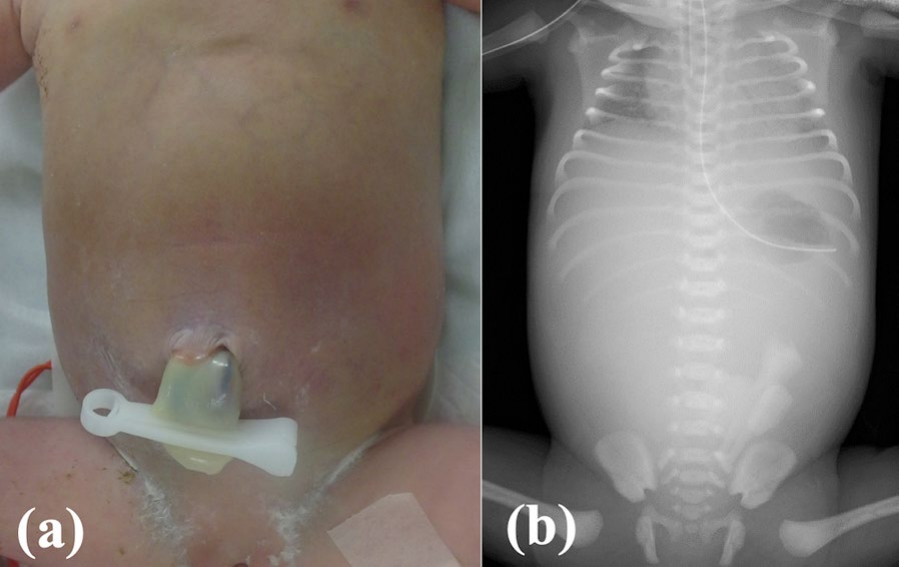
General appearance and the X-ray at birth: a newborn’s abdomen was dark and severely distended. b X-ray shows gasless abdomen
